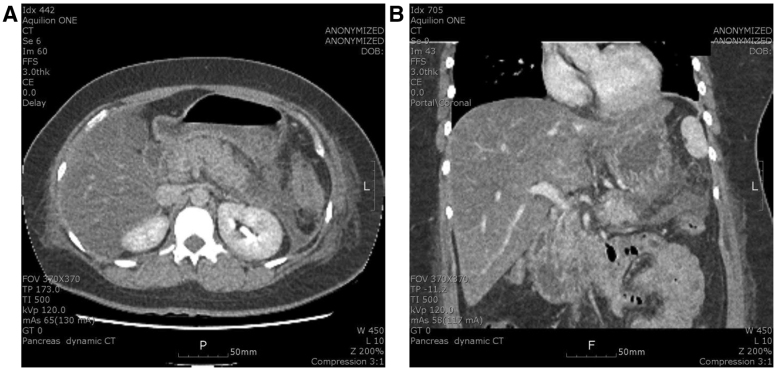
Computed tomography scan. (A) A diffuse parenchymal swelling with peripancreatic infiltration can be seen. Fluid collection with subtle peripheral enhancement is observed around the tail portion of the pancreas, peri-splenic space, and left anterior pararenal space. Furthermore, a heterogeneously decreased parenchymal enhancement can be seen in the distal body and tail portion of the pancreas. (B) Hypo-attenuated liver indicates the possibility of severe fatty liver or toxic hepatitis. CE, CT = computed tomography, FFS, FOV, GT, Im, Idx, kVp, mAs, Se, TI, TB.
